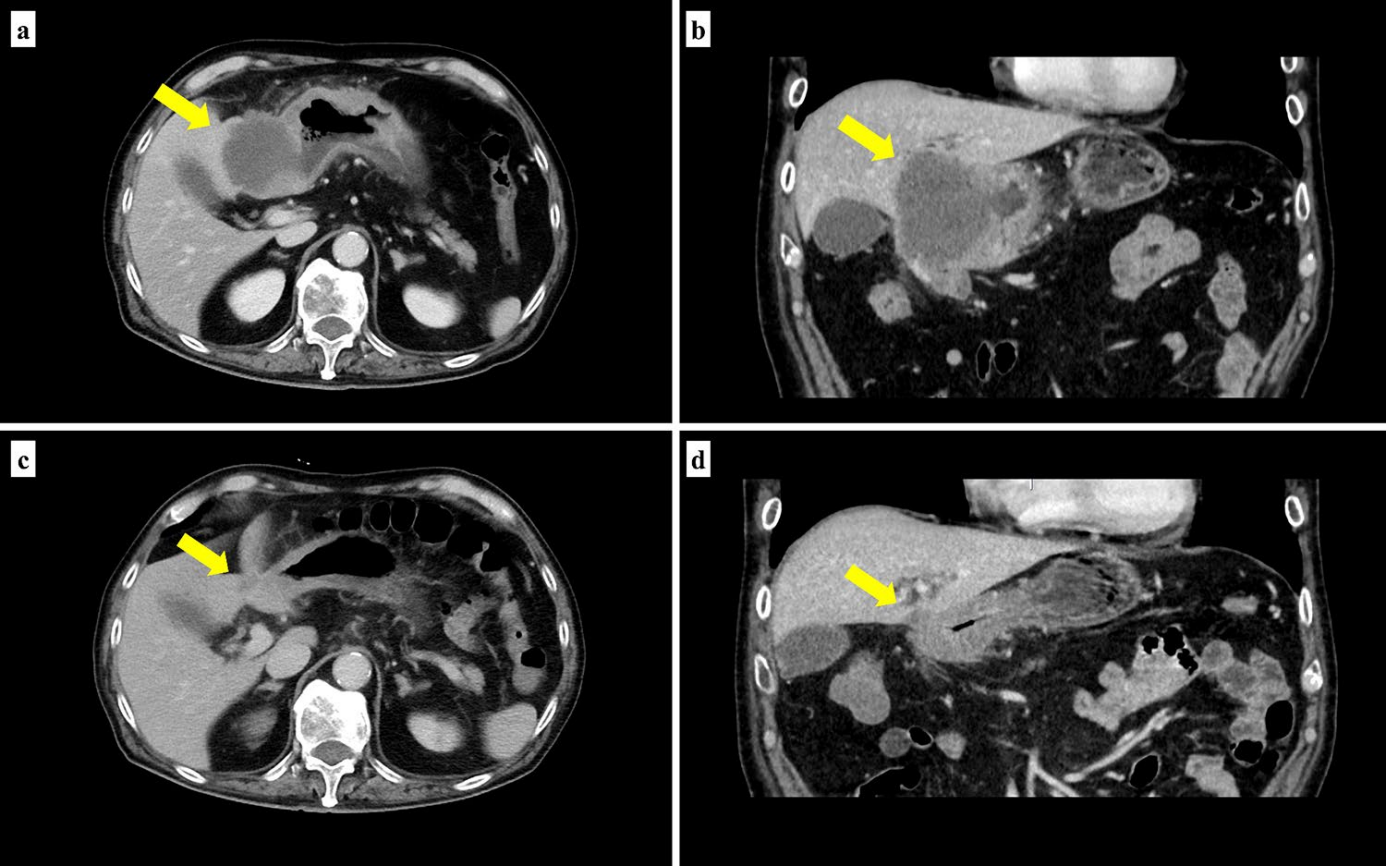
Contrast-enhanced computed tomography. a, b Before chemotherapy (yellow arrows: primary tumor, hilar lymph nodes, and S4 segment of the liver were involved). c, d After three cycles of SOX + Tmab
